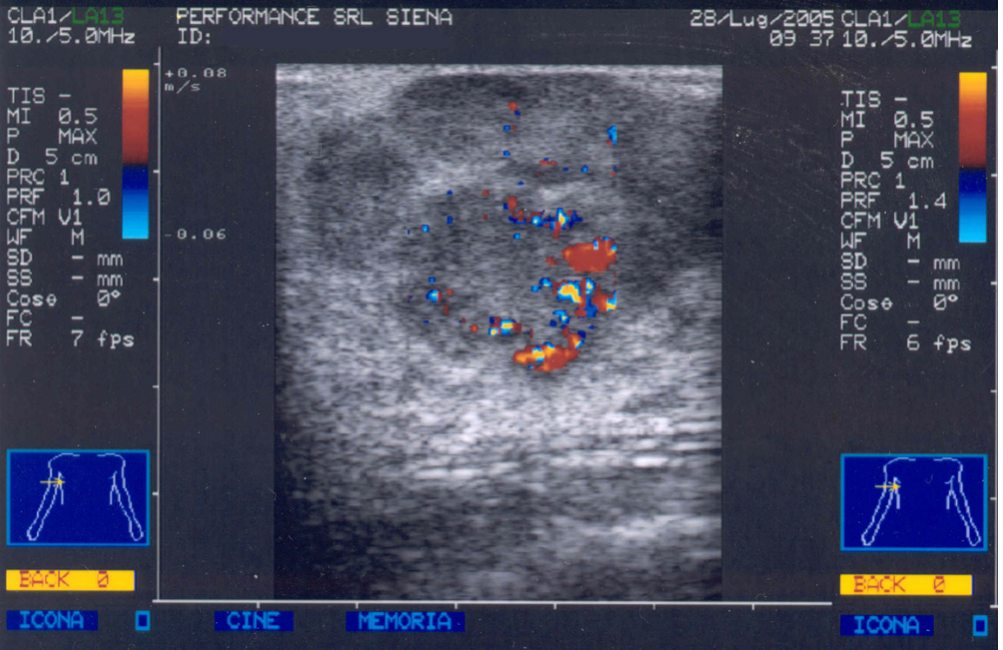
Breast ultrasonography (US) shows the presence of a hypervascularized non-homogeneous mass with ill-defined margins.
